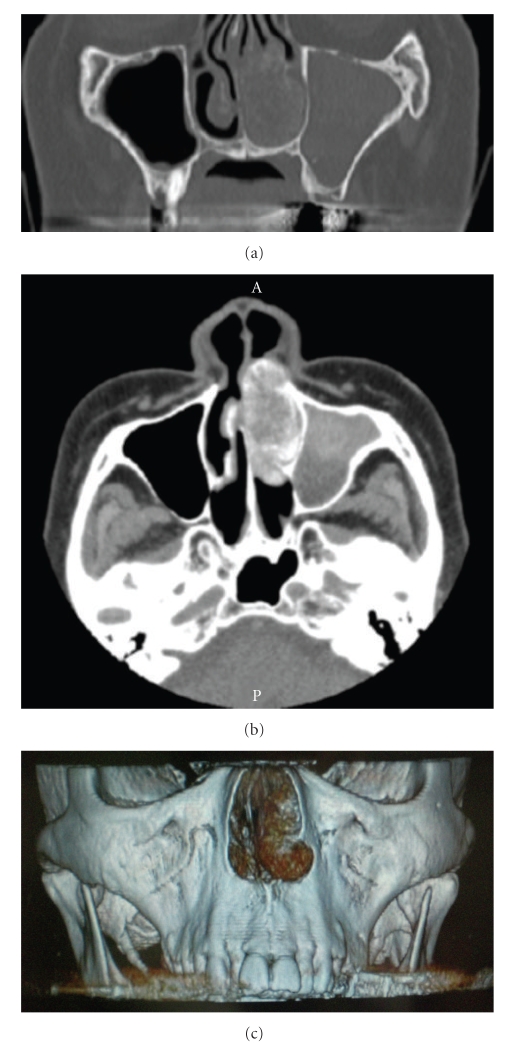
Computed tomography ((a): coronal section, (b): axial section and (c): three-dimensional reconstruction) showing the mass of the inferior turbinate that filled the nasal cavity.
